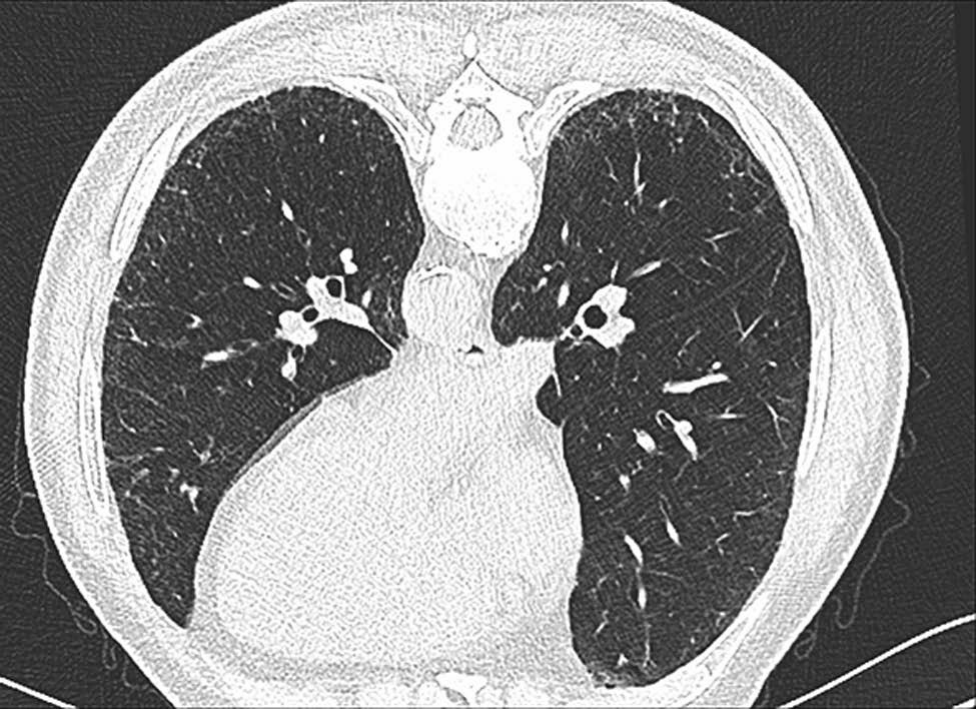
Representative CT image of GGO. M, 71yR, black arrow- GGO in subpleural area of lower lobe of left and right lung. CT = computed tomography, GGO = ground-glass opacity.
